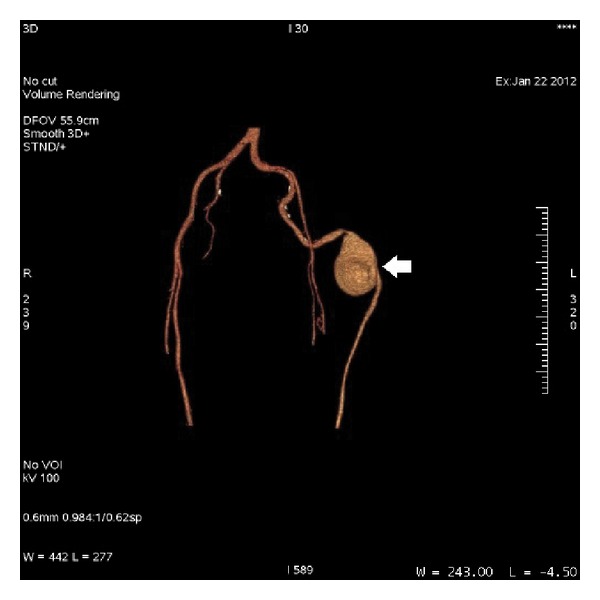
CT angiogram. The arrow shows the complete type of persistent sciatic artery with aneurysmal dilatation.
